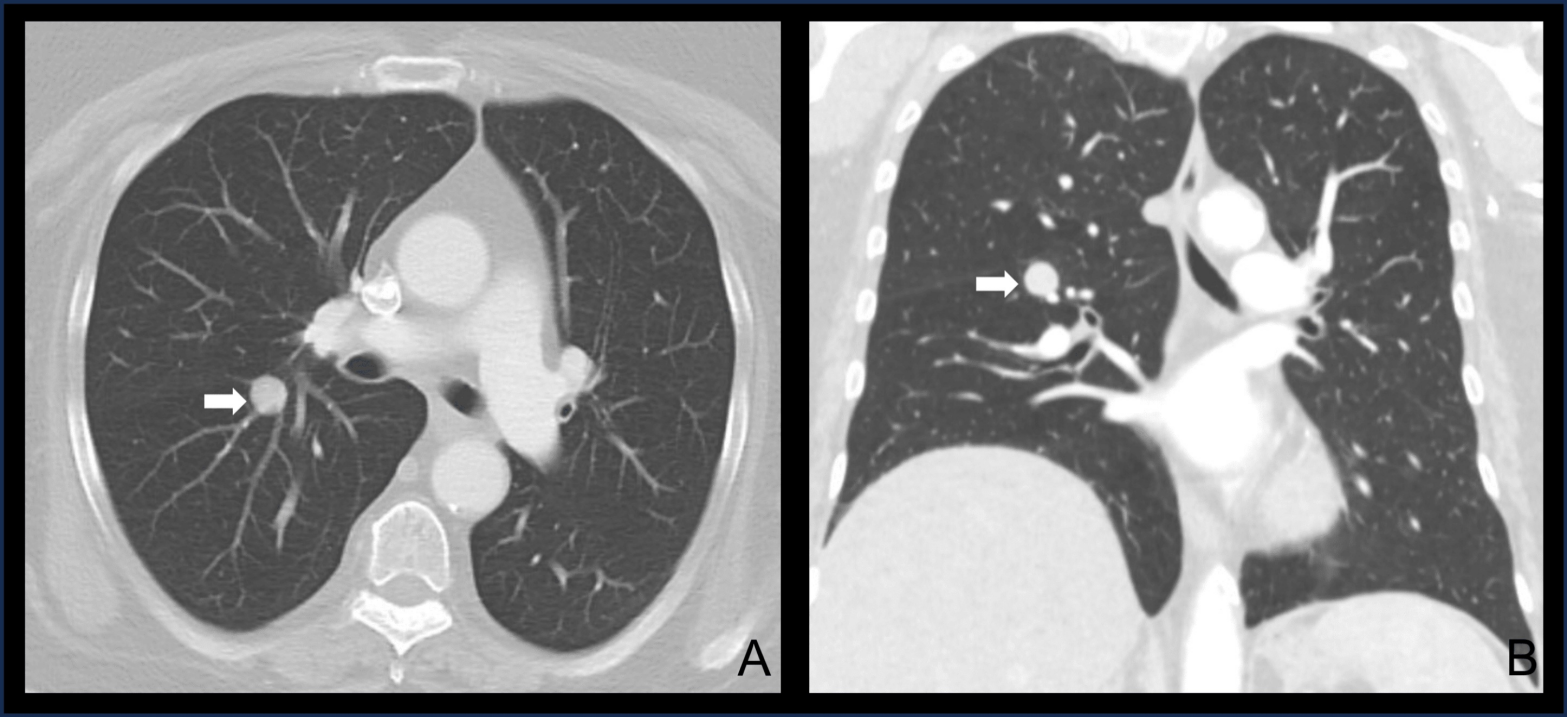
Pre-operative computed tomography scan of the chest A computed tomography scan demonstrating an axial (A) and coronal (B) view of a round, perihilar mass located within the patient’s right lower lobe (white arrow).
